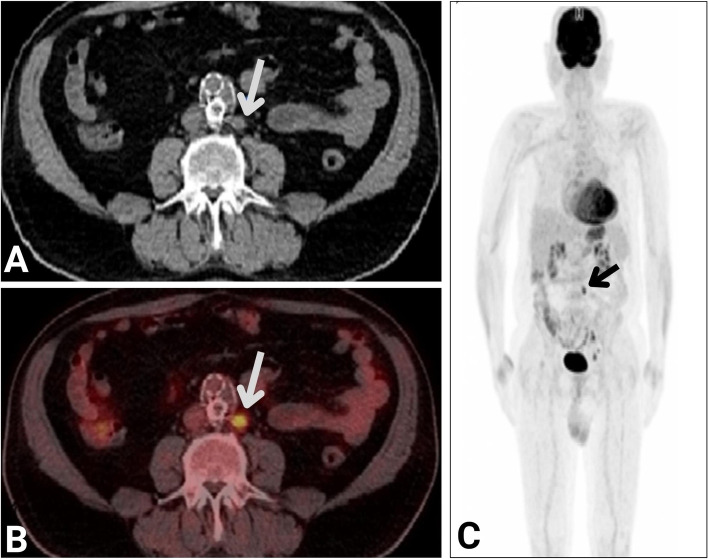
A 73-year-old patient diagnosed with bladder cancer who underwent ^18^F-FDG PET/CT for staging. Axial CT scan (a) showed 6 mm retroperitoneal lymph nodes (white arrow) that was not considered suspicious based on CT scan morphologic criteria. Axial fused PET/CT images (b) demonstrated the hypermetabolic retroperitoneal features of this small lymph node, denoting its metastatic nature (white arrow). MIP PET (c) shows the hypermetabolic abdominopelvic lymph nodes (black arrow)
